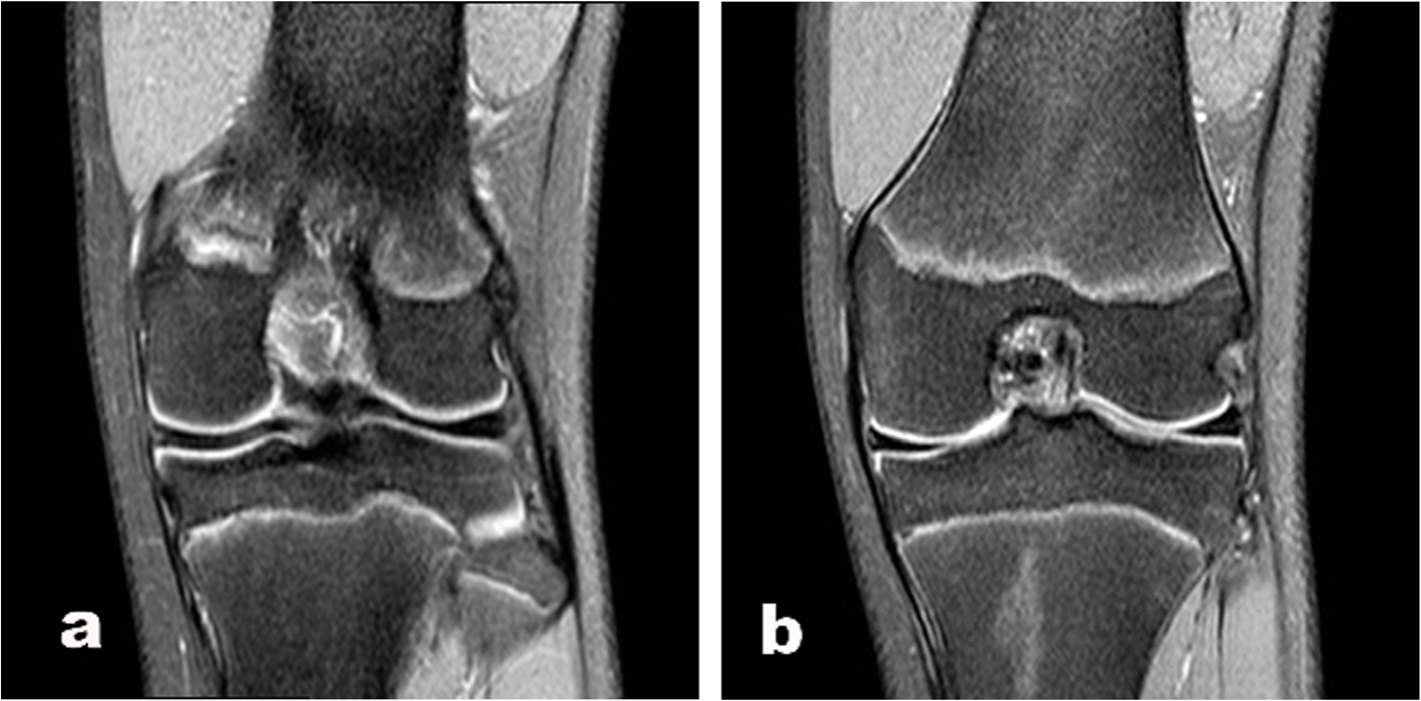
Coronal T2-weighted MRI scan shows absence of the posterior medial root (A) and on the coronal image no clear signs of extrusion were detected (B)
